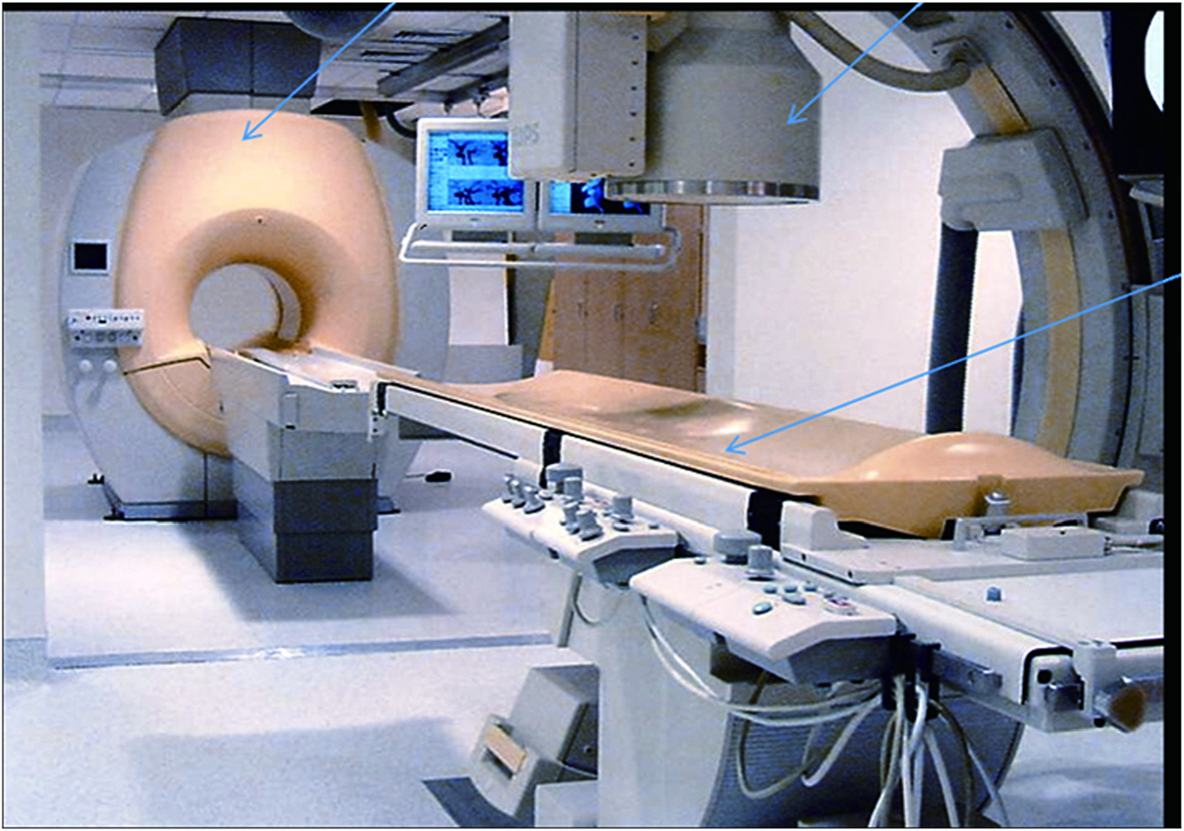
XMR suite: arrows indicating (from left to right) the MRI, fluoroscope, and sliding table. The glass door between the MRI and the sliding table can also be seen.
